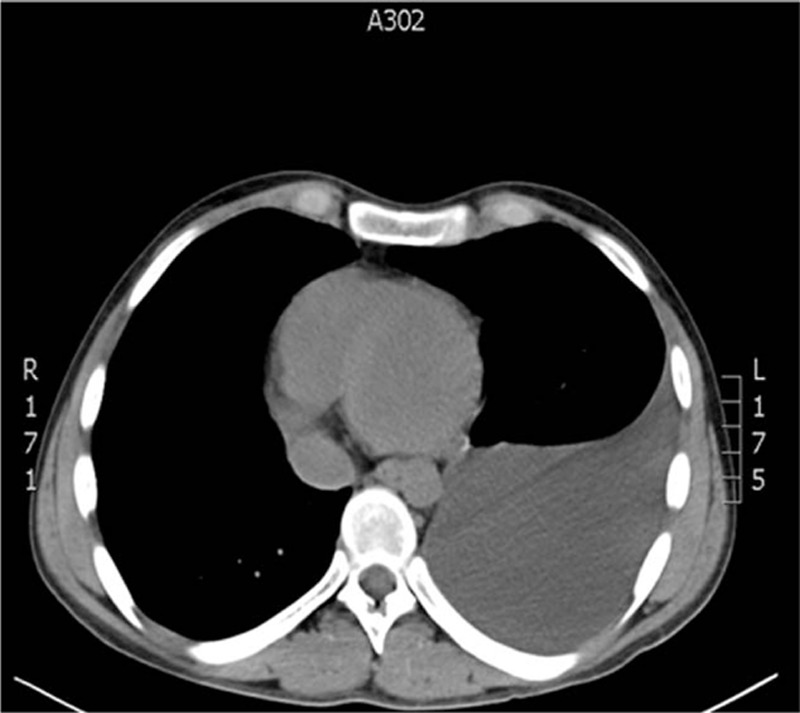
Control computed tomography after chemotherapy—massive pleural effusion in left pleural space.
